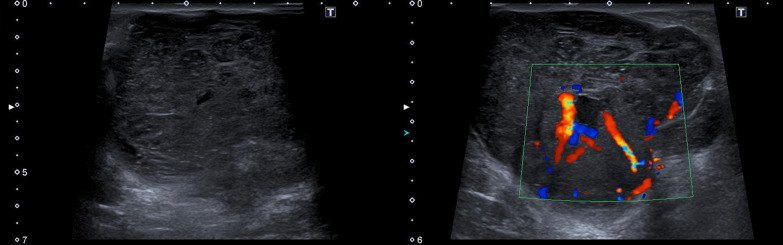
A Ultrasonographic and B Color Doppler examination demonstrates hypoechoic areas which represent cystic areas and increased vascularization of the tumor
